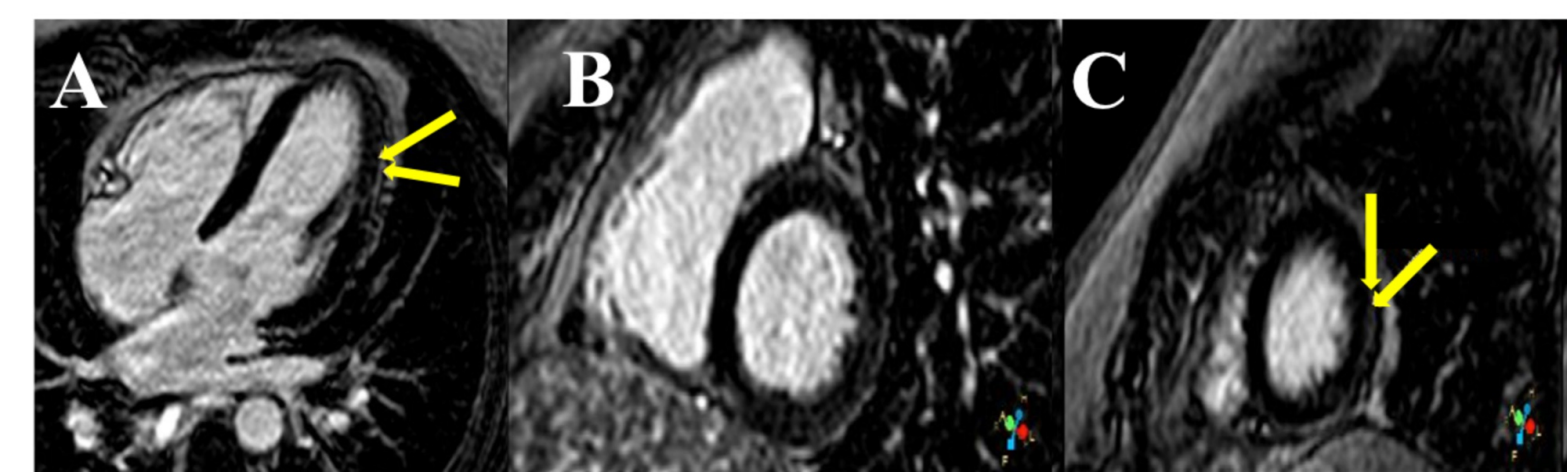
Late gadolinium enhancement cardiac magnetic resonance images: subepicardial delayed enhancement at the lateral left ventricular wall (arrows) (A) Long-axis view. (B) Short-axis view, basal segment. (C) Short-axis view, apical segment
